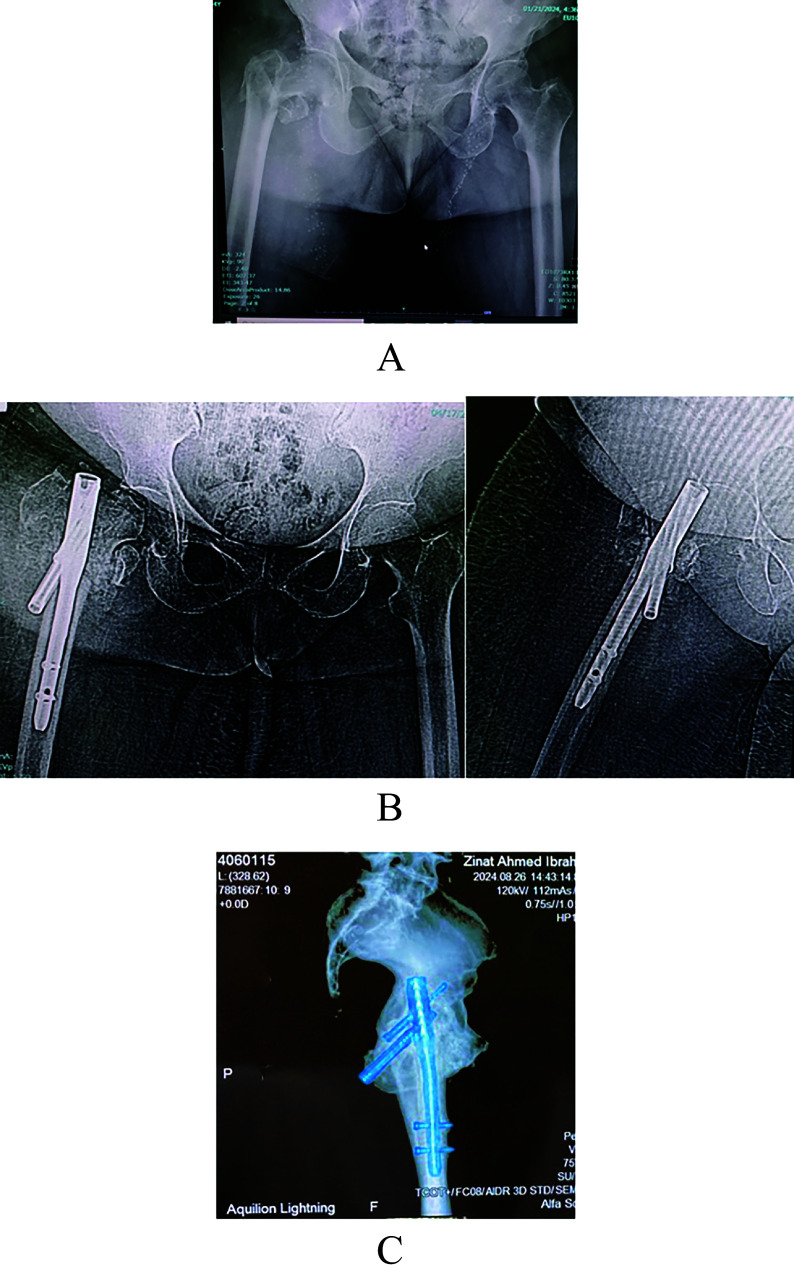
(A) (AO 31–A2.3) fracture pattern, (B) AP and lateral views 2 m later post-surgery showing AVN and varus collapse, and (C) CT 3D showing z effect.
